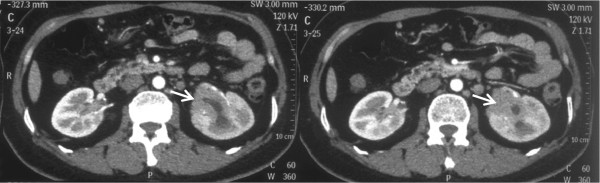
Abdominal computed tomographic scans. These scans show a low-density renal pelvic mass (white arrows) and hydronephrosis of the left kidney.
